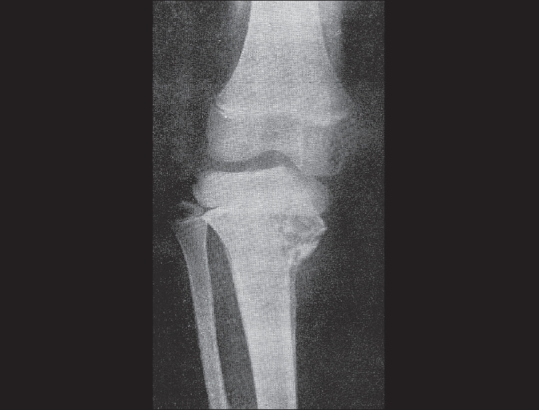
Ewing's sarcoma of the proximal tibia. The plain radiograph shows an irregular, osteolytic lesion in the medial aspect of the proximal tibial metaphysis. This was suspected to be osteomyelitis, but turned out to be a Ewing's sarcoma on biopsy
